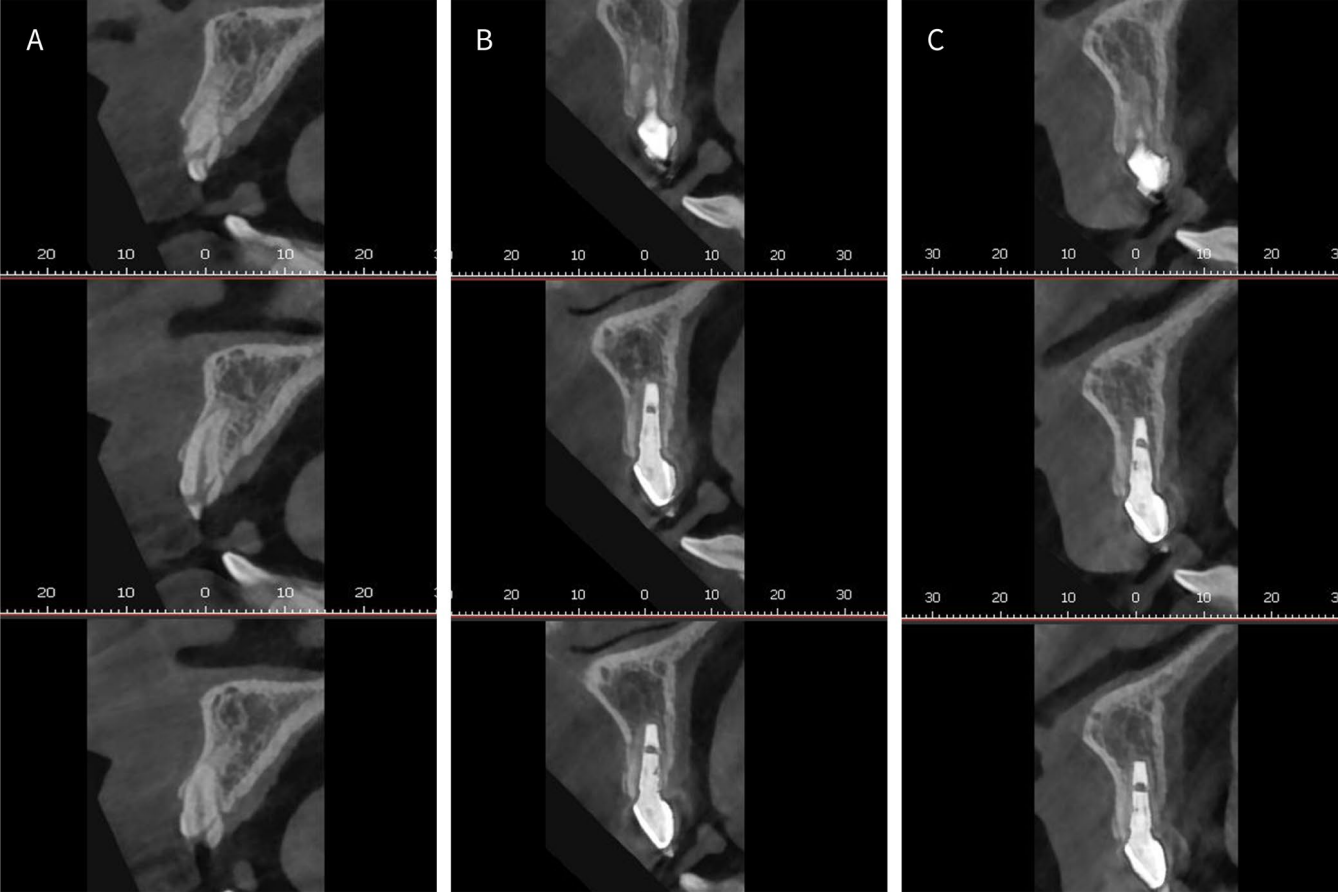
(A) Preoperative CBCT scan; (B) Final restoration CBCT scan; (C) Two‐year follow‐up CBCT scan.
